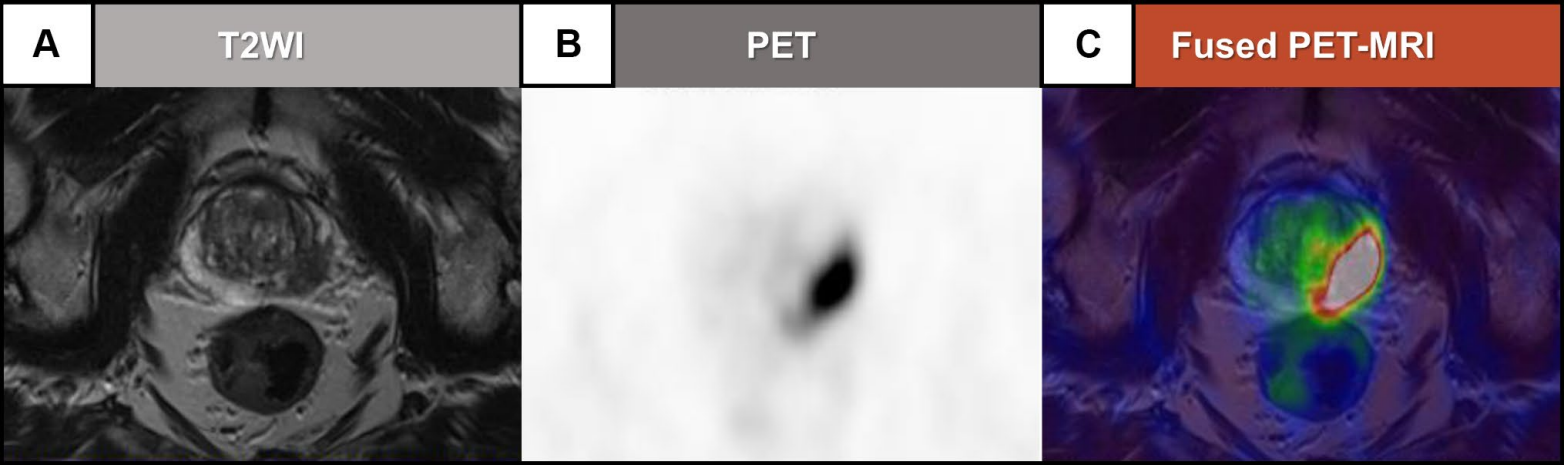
A 73-year-old man with lower urinary tract symptoms and elevated PSA levels (last one 3.7 ng/mL). Patient had a history of two previous negative transrectal biopsies, the last one performed one year before the ^68^ Ga-PSMA PET/MRI examination. The patient was submitted to a prostate ^68^ Ga-PSMA PET/MRI examination, in which T2 weighted-images (A) revealed a hypointense area in the left peripheral zone. PET images (B) demonstrated high PSMA tracer uptake in the same topography of the hypointense area on T2 weighted-images, as confirmed by fused ^68^ Ga-PSMA PET/MRI image (C). Image courtesy of Dr. Marcelo Livorsi da Cunha and Dr. Ronaldo Baroni from Albert Einstein Israelite Hospital, Sao Paulo, Brazil
